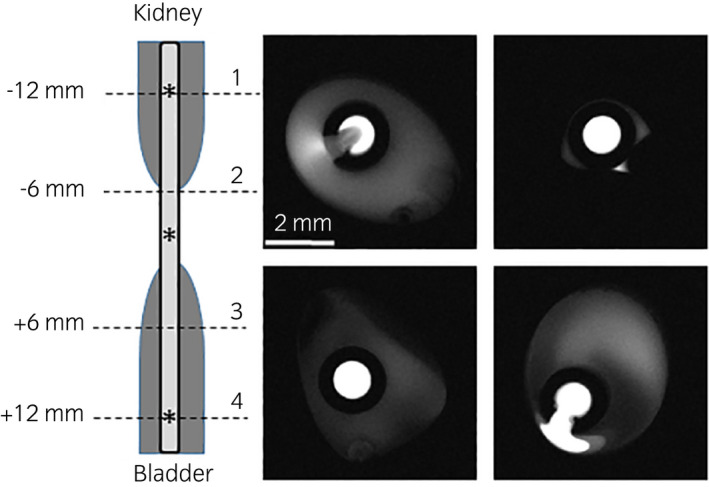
Representative MRI cross‐sections showing flow through side holes proximal (1) and distal (4) to the EUO (2, 3). Images are for a single 6F stent. Images (1) and (4) show urine velocities in stent and ureter lumina, and as well within the connecting stent side hole; images (2) and (3) show relative urine velocities in the stent lumen, which in this region conduct 100% of the flow. *Indicates location of a stent side hole. [Colour figure can be viewed at wileyonlinelibrary.com]
